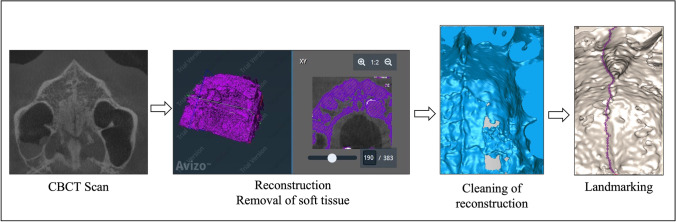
Process of data preparation and analysis. Note: The figure shows, from left to right, CBCTs viewed in RadiAnt DICOM Viewer, segmentation of bony structures in Avizo, processing of three-dimensional surfaces in Geomagic and landmarking of two-dimensional photographs using R packages. Abbreviations: CBCT, cone-beam computed tomography
